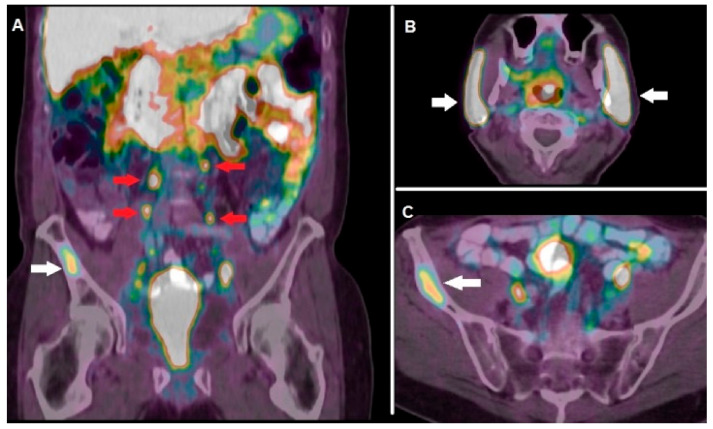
A 74-year-old patient affected by mCRPC (Gleason score 5 + 4), progressing after surgery, androgen deprivation therapy (ADT) and chemotherapy, submitted to PET/CT with ^68^Ga-PSMA-11 before enrollment for ^177^Lu-RLT. Coronal fused images (A) showed highly increased tracer incorporation in abdominal lymph nodes (red arrows) and in the right iliac bone (white arrow). Note the intense physiological radiopharmaceutical uptake in salivary glands (B), white arrows), while fused axial image of the pelvis well demonstrates ^68^Ga-PSMA-11 uptake in the iliac metastasis (C) white arrow).
